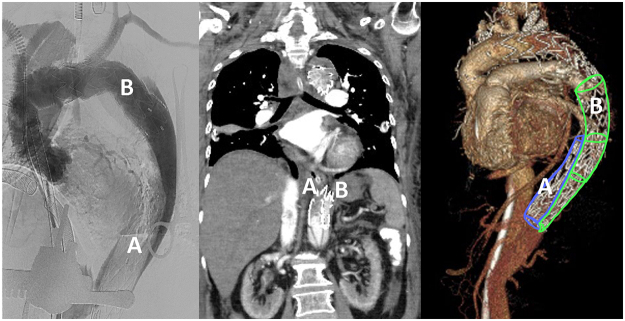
Angiography occasionally led to misidentified stent positions, suggesting stent A in the FL and stent B in the TL, which led to stent B being extended during surgery. However, postoperative CT revealed the error (middle panel), significantly aiding decision-making and improving patient outcomes.
